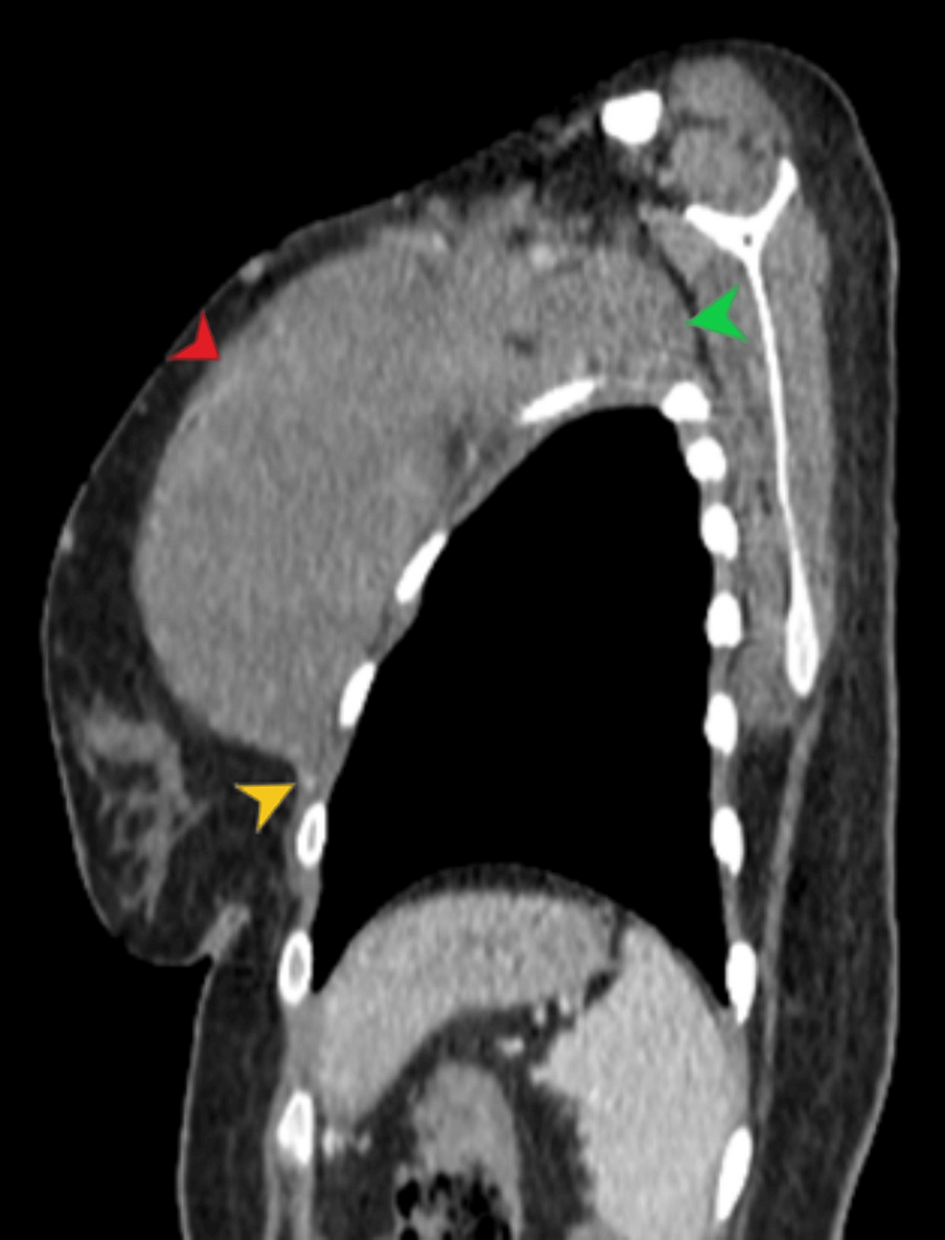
Sagittal post contrast CT chest demonstrates a well-circumscribed soft tissue density lesion annotated by red arrow head. The lesion is inseparable from underlying chest wall muscles (yellow arrow head). There is extension into the left axilla (green arrow head).
